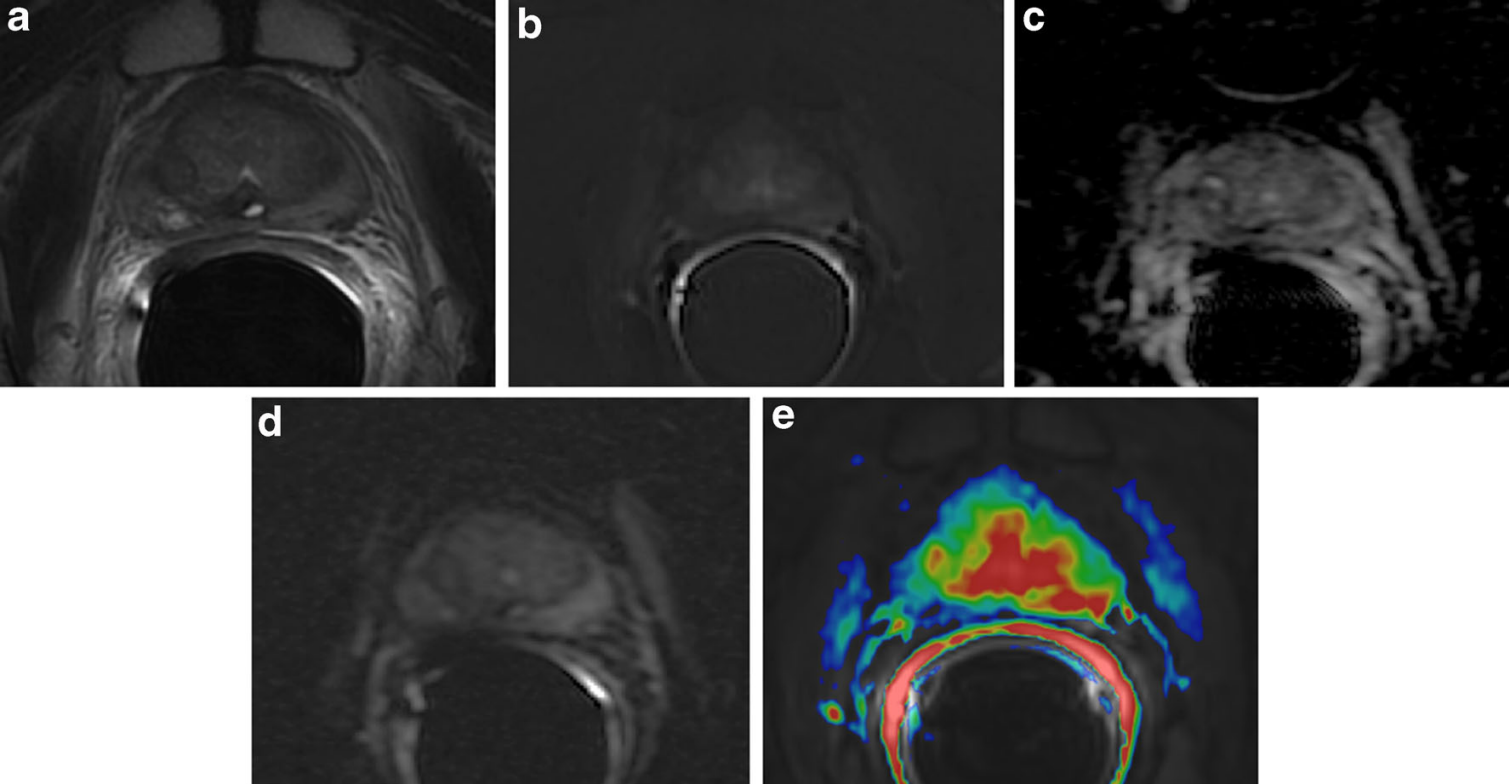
Post-RT treatment. a Axial T2-weighted fast spin-echo image showing an area of intermediate signal intensity in the peripheral posterior left portion of the gland after 3 months post-RT treatment suspected for recurrence. b Axial perfusion gradient-echo T1-weighted image shows mild enhancement in the suspected area. c ADC map and d axial DWI image with b value 1000 mm^2^/sec showing no areas of suspected restriction of water diffusivity. e colour DCE MR map showing marked enhancement in posterior left peripheral zone, corresponding to post-RT outcomes
